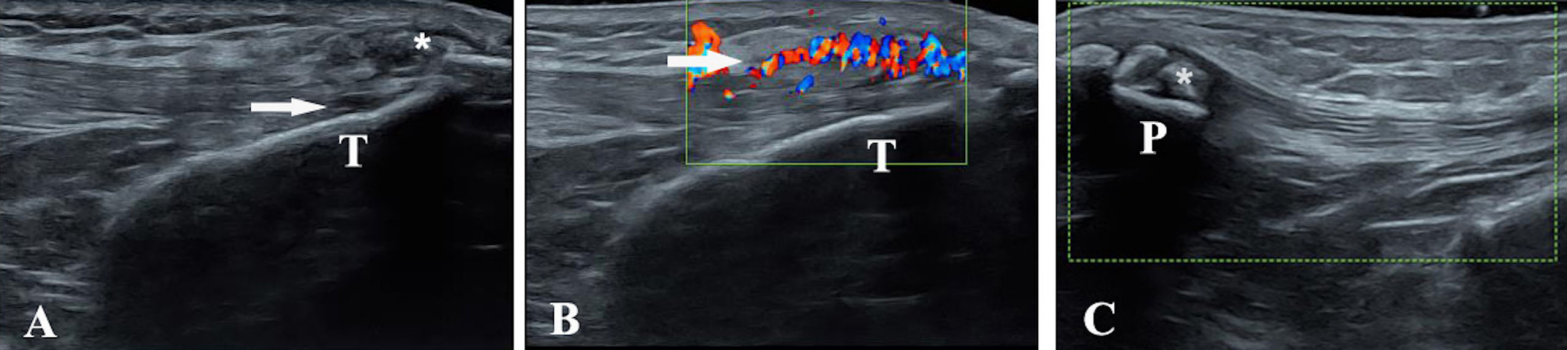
Ultrasound evaluation in gouty patients. Longitudinal scans of the patellar ligament and the quadriceps tendon in B-mode and power Doppler ultrasound. (A) The asterisk shows the tophus of the patella ligament. (B) Doppler flow signals indicate acute inflammation of quadriceps tendon. (C) The asterisk shows the tophus of the quadriceps tendon.
